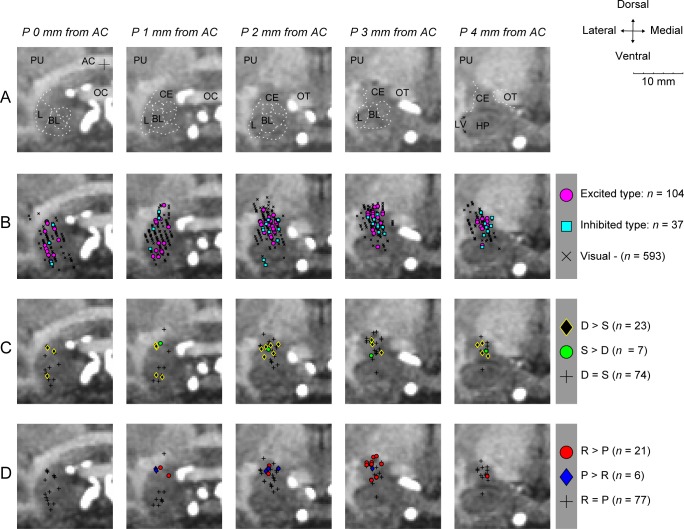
Estimated positions of recorded neurons. Recording sites are shown in five coronal MR images spanning 0–4 mm posterior to the AC. (A) Amygdala and surrounding brain areas. (B–D) The neurons’ positions are shown, based on different features. (B) Neurons excited and inhibited by visual scenes during the fixation period, and neurons showing no response to the scenes (Visual −). (C) Excited-type neurons showing significantly different activity between dangerous (D) and safe (S) contexts (D > S; S > D), and others (D = S). (D) Excited-type neurons showing significantly different activity between rich (R) and poor (P) contexts (R > P; P > R), and others (R = P). AC, anterior commissure; BL, basolateral complex of the amygdala; CE, central nucleus of the amygdala; D, dangerous; HP, hippocampus; L, lateral nucleus of the amygdala; LV, lateral ventricle; MR, magnetic resonance; OC, optic chiasm; OT, optic tract; P, poor; PU, putamen; R, rich; S, safe.
